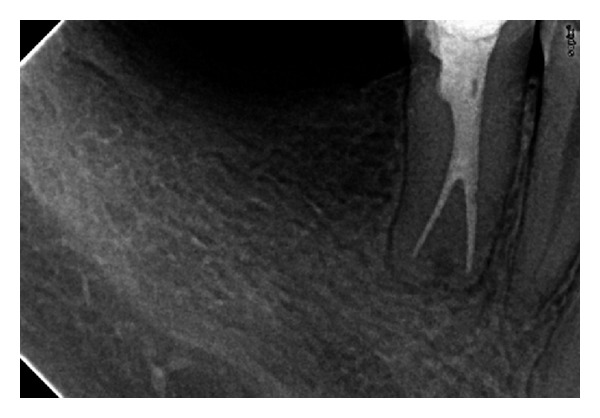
Periapical radiograph showing obturated canals.
